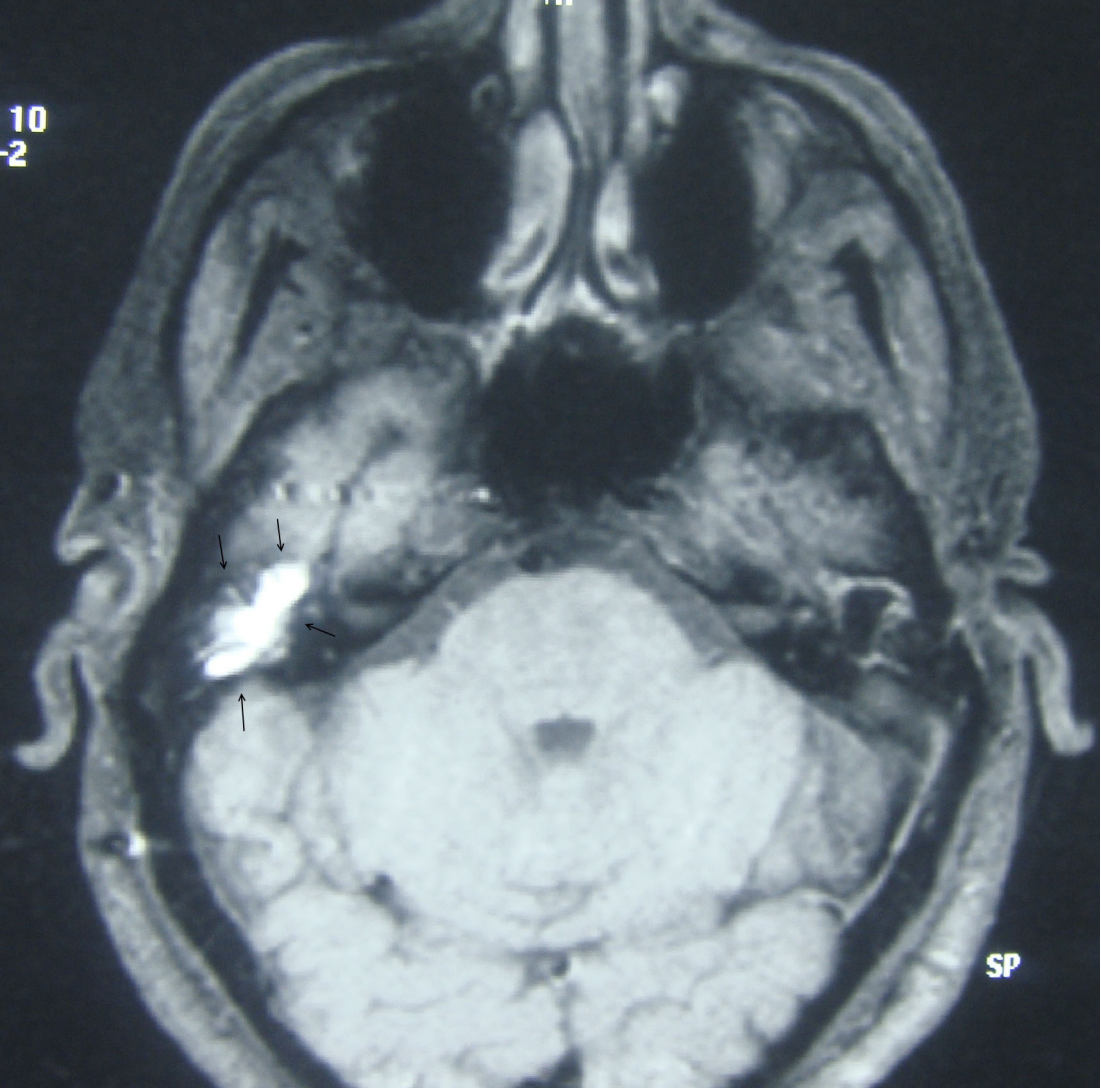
T1-weighted temporal bone MRI showing a homogenous mass with increased signal intensity relatively to the brain. Arrows indicate the mass.
